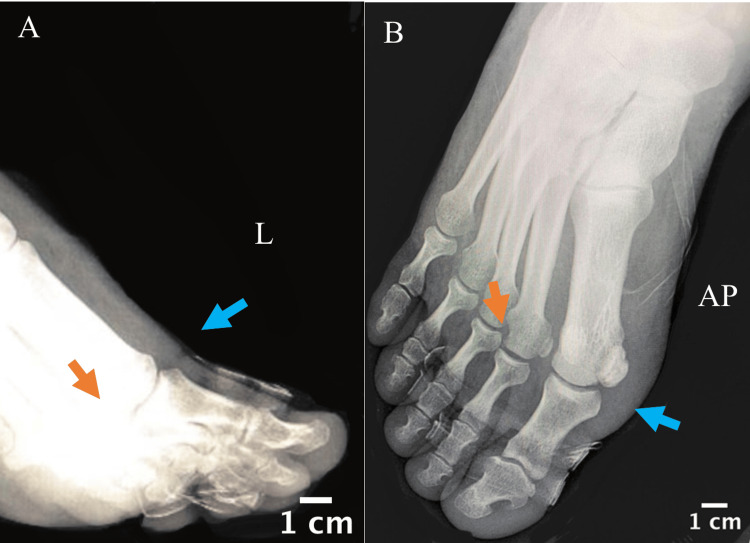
X-rays lateral (A) and anteroposterior (B) views after closed reduction of the first toe dorsal dislocation under general anesthesia followed by buddy taping; arrows point to the reduced first (blue arrow) and second (red arrows) MTP joints. MTP: Metatarsophalangeal.
